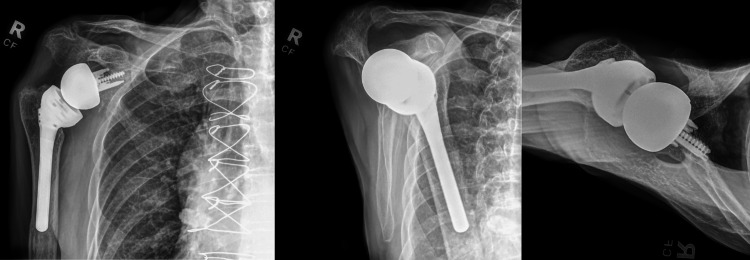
Radiographs of the right shoulder at the 9.5-week postoperative visit demonstrating well-aligned revision reverse shoulder arthroplasty implants
